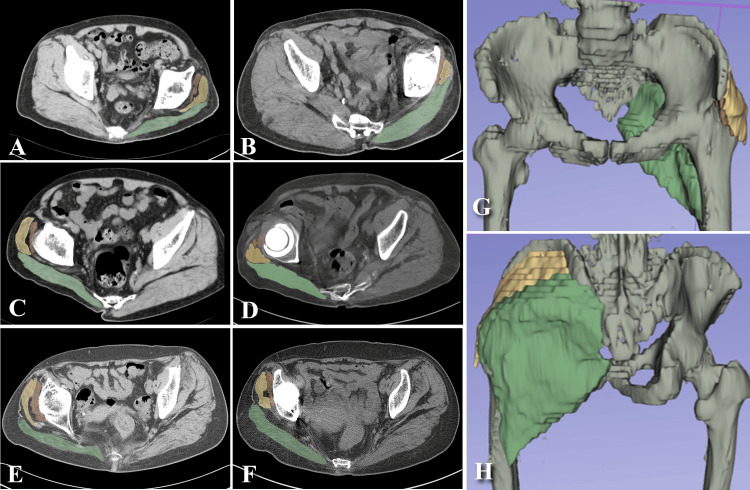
Measurement of muscle volume around the hip. A, B. Plane CT axial image of Case 1 before and seven years after operation. C, D. Plane CT axial image of Case 2 before and seven years after operation. E, F. Plane CT axial image of Case 3 before and seven years after operation. G, H. 3D images of Case 1 before operation By tracing the cross-sectional areas of the gluteus maximus (green), gluteus medius (yellow), and gluteus minimus (red) on every third slice of the CT images using 3D image analysis software (3D slicer), the volumes of the reconstructed muscles were quantified. Case 1: A 65-year-old man; Case 2: A 73-year-old man; Case 3: A 75-year-old woman
